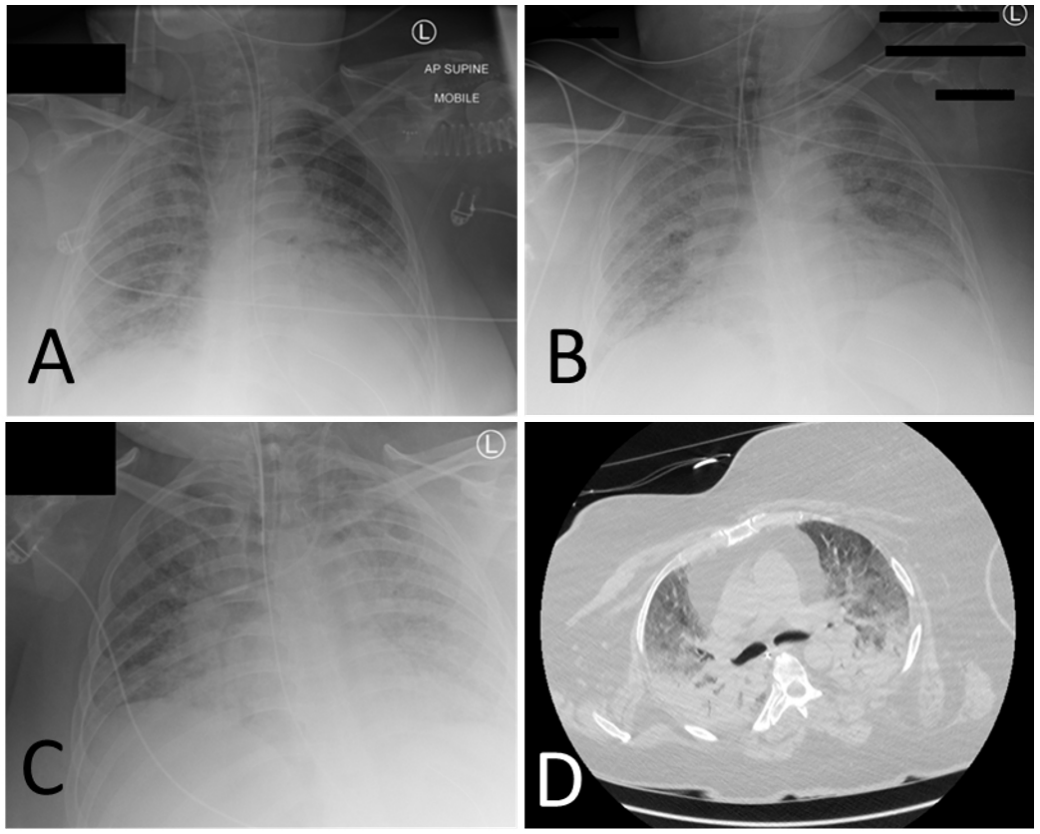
Chest x-rays from A) day 8, B) day 17, and C) Day 30. D) Computed tomography pulmonary angiogram on day 9 demonstrating widespread ground glass appearances consistent with COVID pneumonitis.
